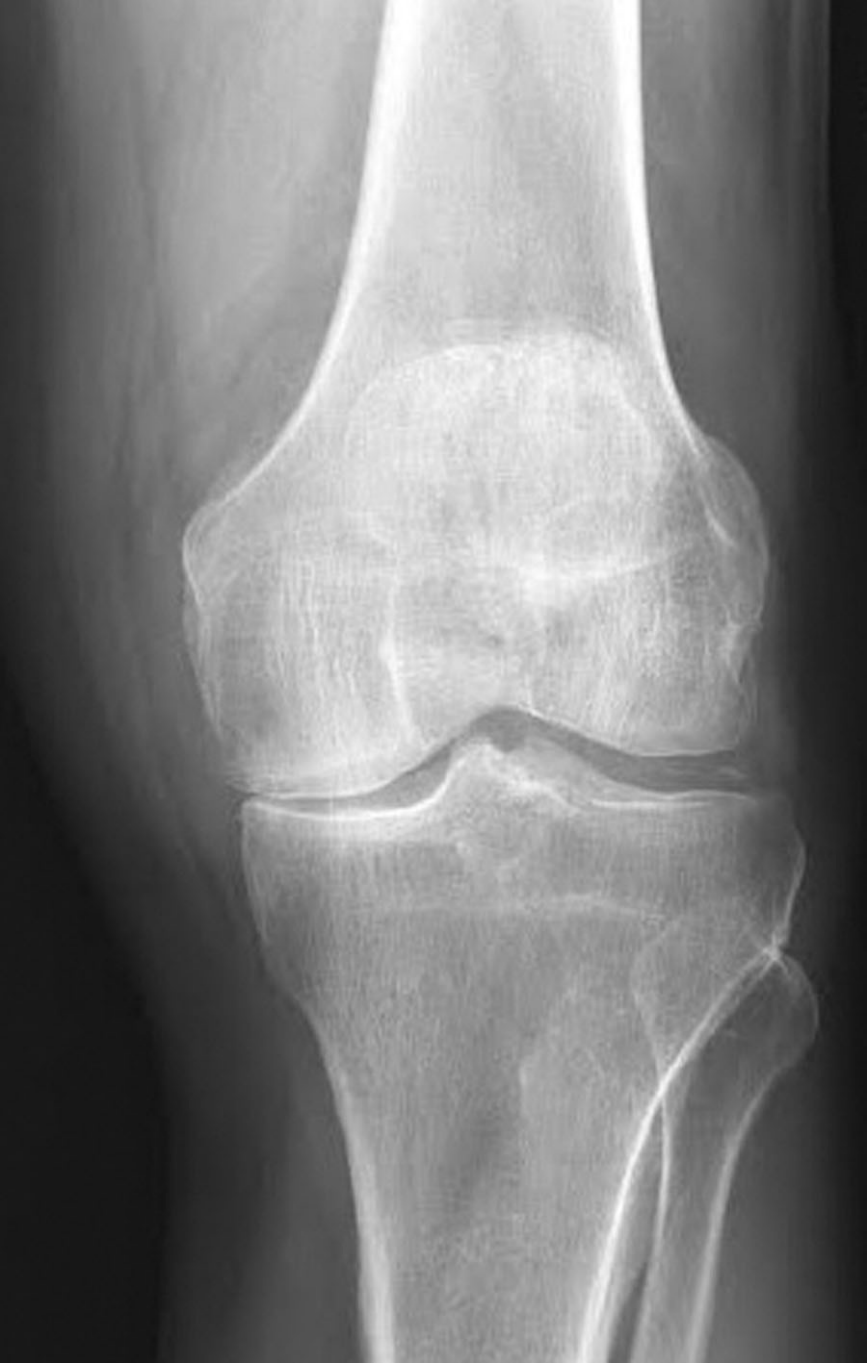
AP radiograph of the left knee
